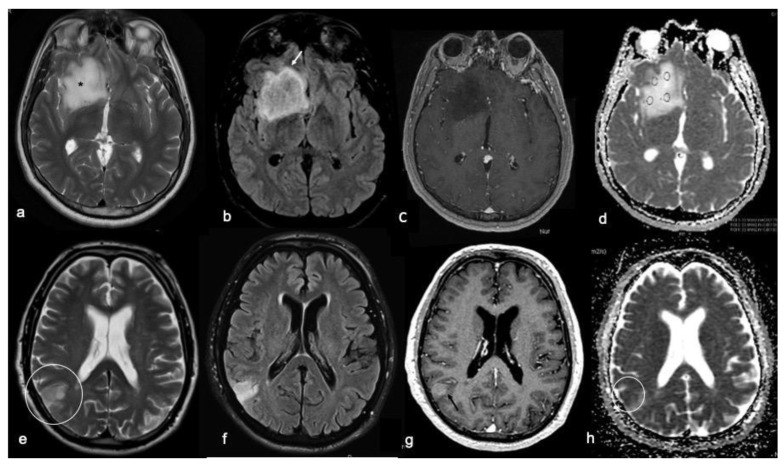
Radiological characteristics of IDH-MUT (top row: (a–d)) and IDH-WT (bottom row: (e–h)) diffuse gliomas. For each case, T2w fast spin echo (a,e), FLAIR sequences (b,f), T1w fast spin echo contrast-enhanced (c,g), and ADC map (d,h) images are shown. (a–d) Right frontal tumor with defined borders, a high and homogeneous T2w signal (asterisk in (a)), a T2w/FLAIR mismatch sign (arrow in (b)), no contrast enhancement, and high ADC values; these are the typical imaging findings of an IDH-MUT glioma. (e–h) Right parietal ill-defined tumor (inside the circle in (e,h)) characterized by a low T2w signal (e), no evidence of a T2w/FLAIR mismatch sign (f), a slight contrast enhancement (g), and a low signal in the ADC maps; these imaging findings are typical of an IDH-WT glioma.
